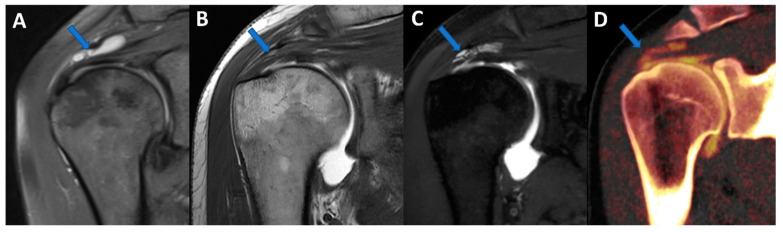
Complete supraspinatus tendon diagnosed using DECTA. On the coronal standard STIR MRI image (A), a fluid collection located on the bursal side of the supraspinatus tendon can be identified (arrow). On the coronal T1 weighted TSE MRA image (B), the supraspinatus tendon appears irregularly thinned, as in a case of a partial tear. There is no apparent passage of contrast material on the bursal side (arrow). On the corresponding 1 mm PD fat-saturated image of the coronal plane (C), there are still no clear signs of complete tendon tears (arrow). The reconstructed DECTA 1 mm coronal image (iodine map; (D)) clearly demonstrates the presence of a complete tear with the passage of contrast material on the bursal side of the tendon (arrow).
